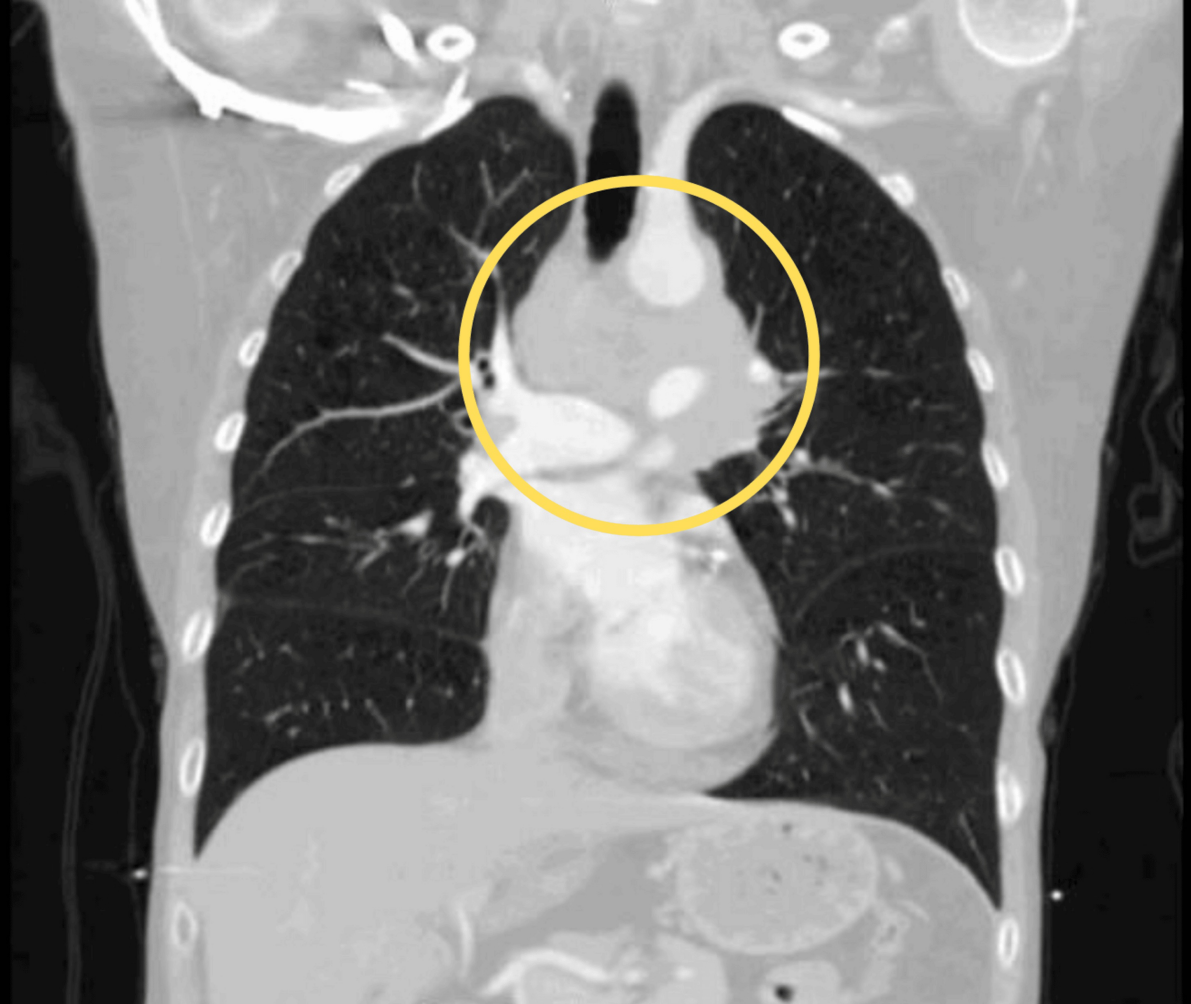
Coronal CT scan showing a precarinal mass (encircled in yellow) accompanied by multiple enlarged lymph nodes in the aortopulmonary window. CT: Computed tomography
